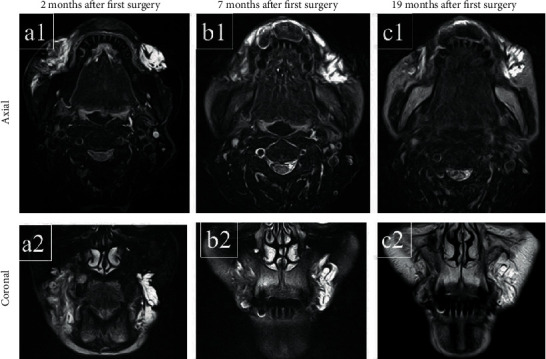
MRI findings. (a1, a2) Axial and coronal images of T2-weighted image of fat suppression two months after the first surgery. Irregular high signal intensity areas under the subcutaneous tissue on both cheeks and high signal intensity areas were widely distributed on the right side. (b1, b2) Axial and coronal images of T2-weighted image of fat suppression seven months after the first surgery. The images showed a reduction of the right nodule compared with those two months after the first surgery. (c1, c2) Axial and coronal images of T2-weighted image 19 months after the first surgery. Unclear boundary lesions showed the high signal area was recognized, and foreign body-like liquid silicone injection was suspected again. The above high signal area on the buccal region was shrinking over time. These findings should develop by loss of silicone from the fistula. The high signal area on the left buccal region had not changed.
